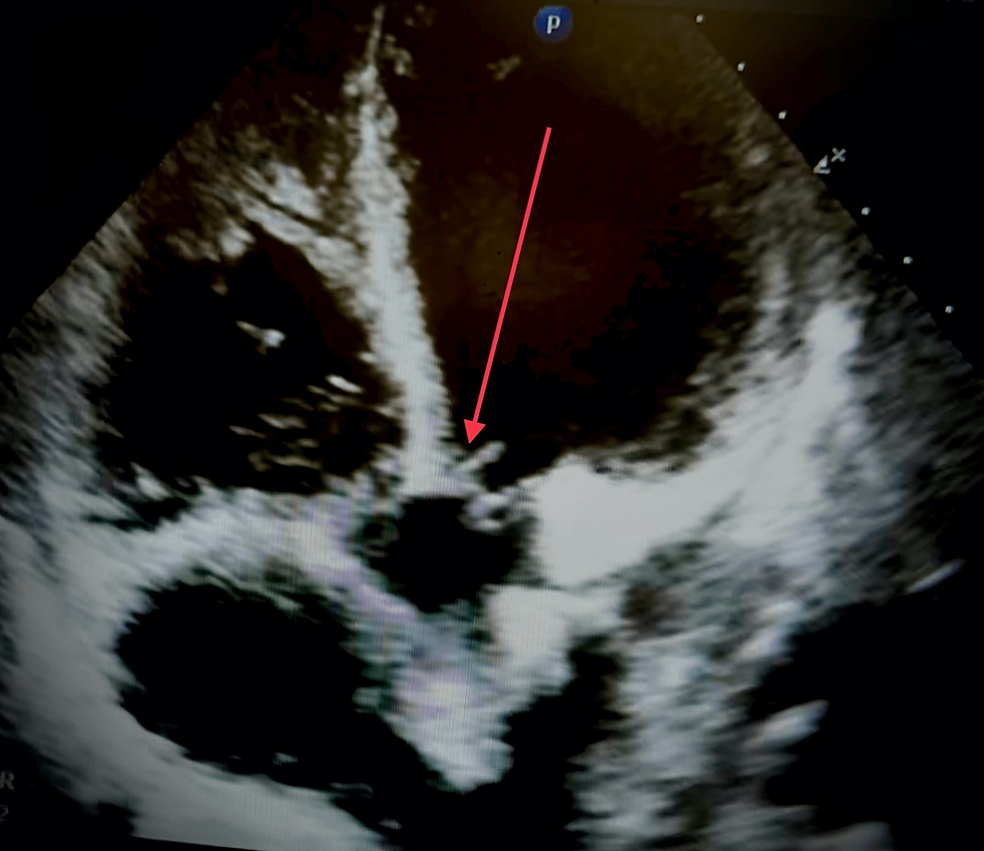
Transthoracic echocardiogram in the four chamber view demonstrating aortic valve vegetation.
